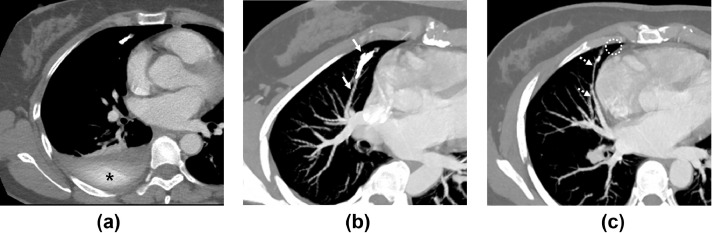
(A) Computed tomography (CT) image 3 d after embolization showing right hemothorax and extravasated contrast medium (asterisk). (B, C) Four-month follow-up CT images with maximum-intensity projection reconstruction showing resolution of all pleural effusions, occlusion of the feeding artery by the embolic devices (arrows), significant reduction in the diameter of the draining vein (dashed arrows), and almost complete disappearance of the venous sac (dashed circle).
